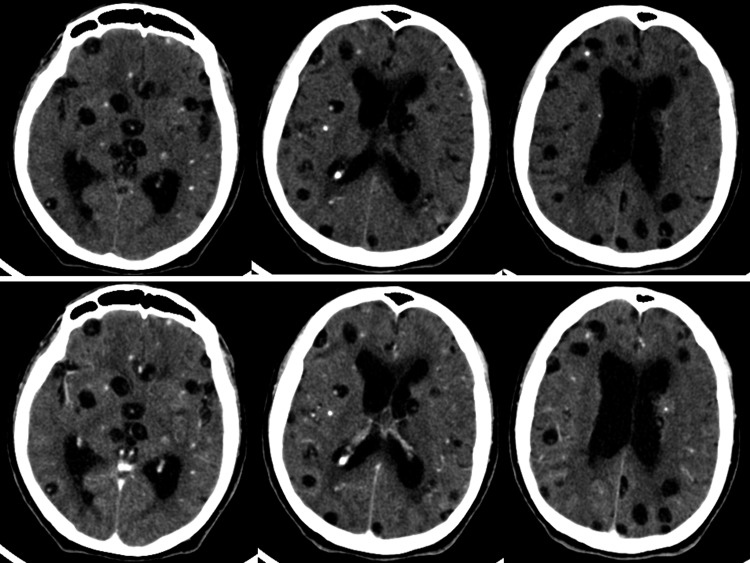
Computed tomography of patient with heavy non-encephalitic neurocysticercosis, showing multiple viable cysticerci and no edema.
